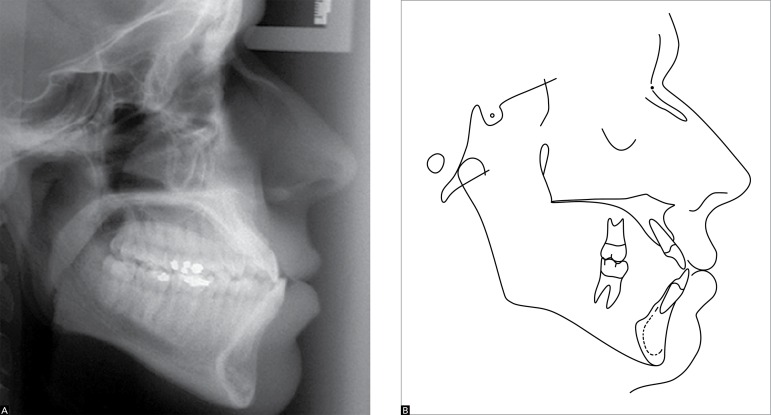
A) Initial cephalometric profile radiograph and B) cephalometric tracing.
